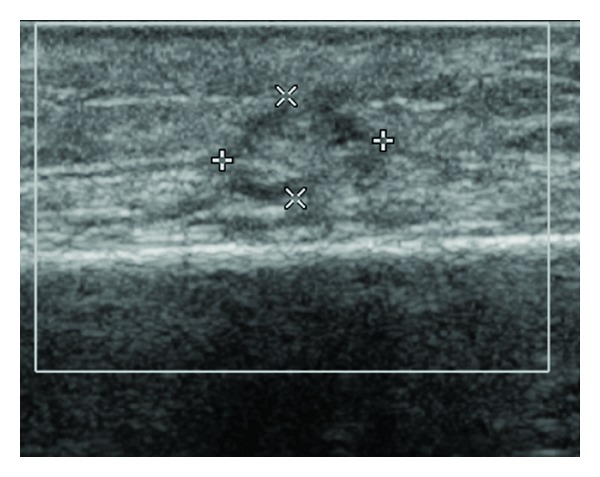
Ultrasound doppler 3 months after trauma. Resolution of the soft tissue edema: hyperechoic (fatty) lesion with hypoechoic halo with relatively clear boundaries. No periosteal reaction was noted.
